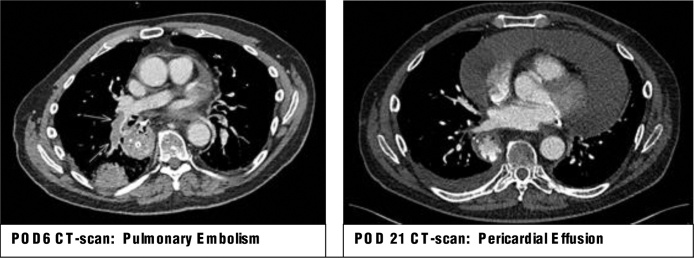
Computed Tomography postoperative Day 6 + Day 21.
